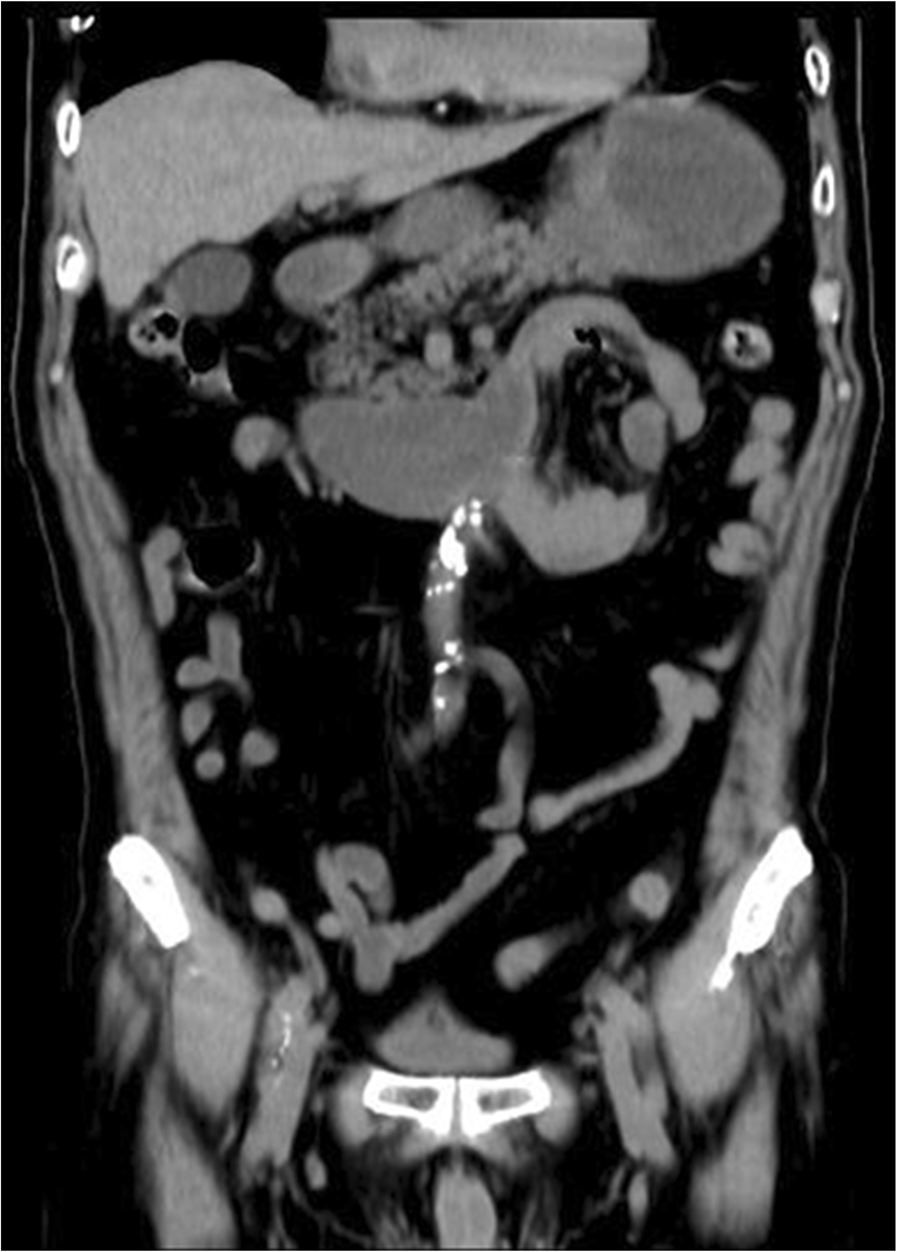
Coronary view of CT scan image showing the cluster of the small intestine
